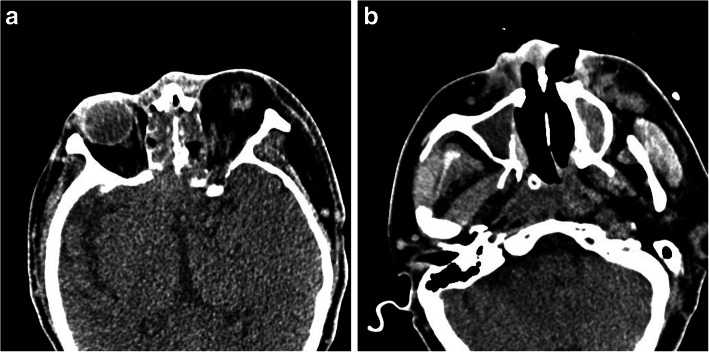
a Computed tomography (CT) scanning of the brain and orbits revealed extensive involvement of the bilateral intranasal sinuses, orbits, extraocular muscles, and soft tissues. Increased soft tissue density was evident in the bilateral maxillary sinus, ethmoid sinus, sphenoid sinus, and around the orbits. Dilatation of the extraocular muscles was also observed, as was erosion of the left lateral wall of the sphenoid and cribriform plate. b Perforation of the patient’s nasal septum affected the left nasal cavity, with evidence of hard palate and left nasal wing involvement and perforation
